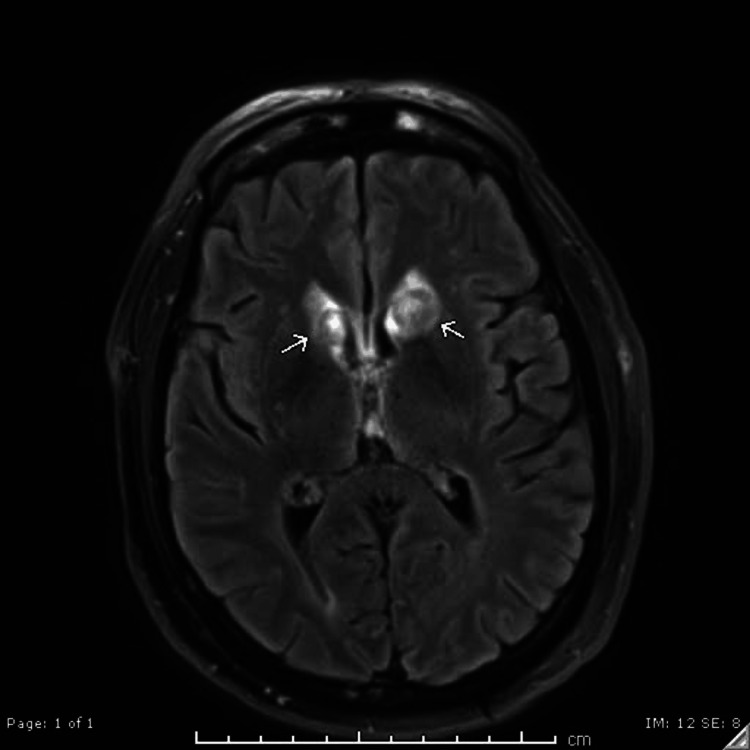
MRI FLAIR sequence 24 days after the original MRI Increased signal abnormalities within the bilateral caudate nuclei. The FLAIR signal within the bilateral caudate lobes appears more heterogeneous with mixed hypointensity and hyperintensity with the rim of hypointensity surrounding a central focus of hyperintensity with an outermost rim of additional hyperintensity. Similar to prior, the left caudate nucleus is more affected than the right with the left measuring 1.5 times the size of the right. Interval development of mass effect from this increased edema resulting in mild mass effect/compression of the frontal horns. Decreased hyperintensities surrounding the bilateral trigones and occipital horns when compared to prior. MRI: magnetic resonance imaging; FLAIR: fluid-attenuated inversion recovery
